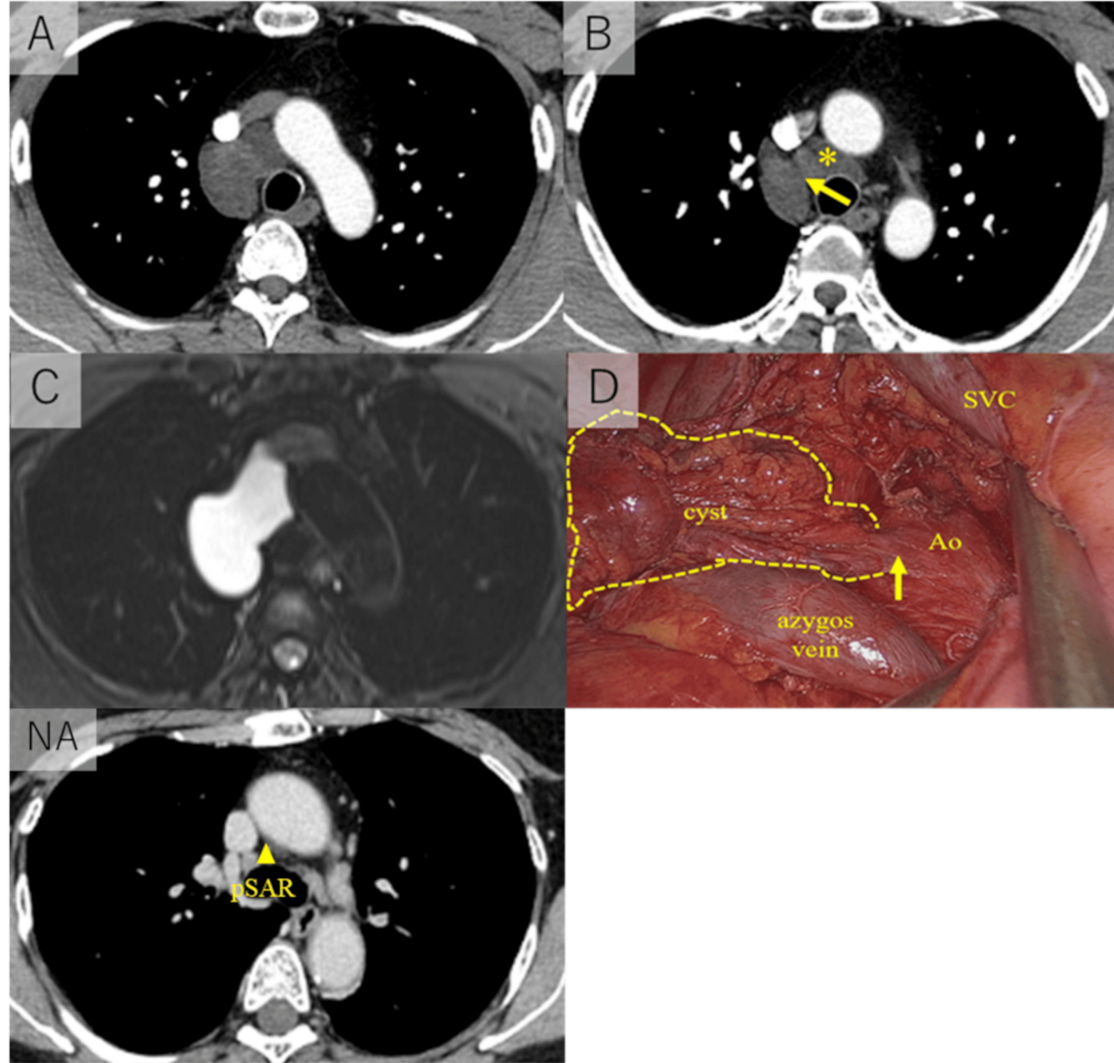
Radiological images and operative findings of Case 1 A: The fluid density mass (5.8 x 3.5 cm) is located in the right paratracheal space of superior mediastinum. B: The origin of the lesion was the fluid collection of the posterior portion of the superior aortic recess (*). The fluid collection extended the cephalad to the right paratracheal space of the superior mediastinum through the yellow arrow route. C: Magnetic resonance image (MRI) of the mass showed a high density on T2-weighted imaging. D: The cystic tumor is connected to the space behind the aorta (yellow arrow = connected part). NA: The normal anatomy figure (reference). The yellow arrowhead is in the pSAR. SVC = superior vena cava; Ao = aorta; pSAR = posterior portion of the superior aortic recess.
